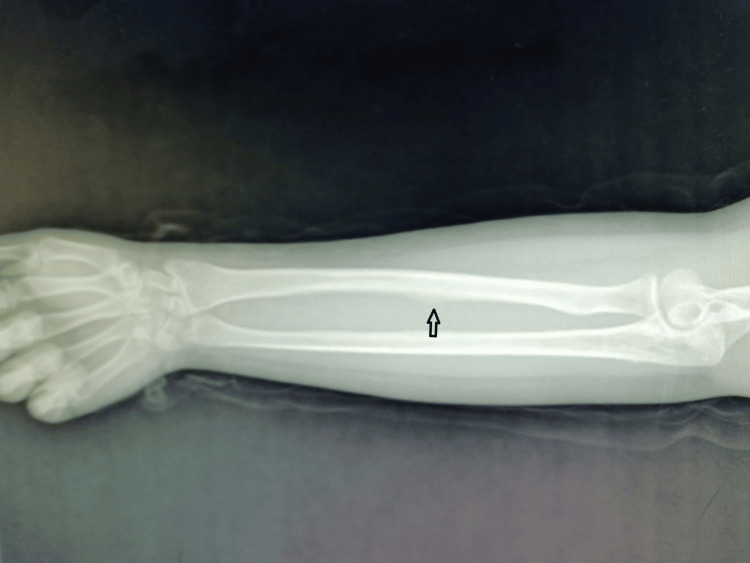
The anteroposterior radiograph of the radius and ulna reveals characteristics consistent with a healed fracture of the ulna (indicated by the arrow).
